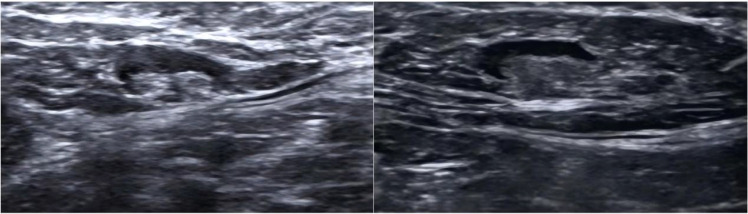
Normal appearance at ultrasound (US) after neoadjuvant therapy.
